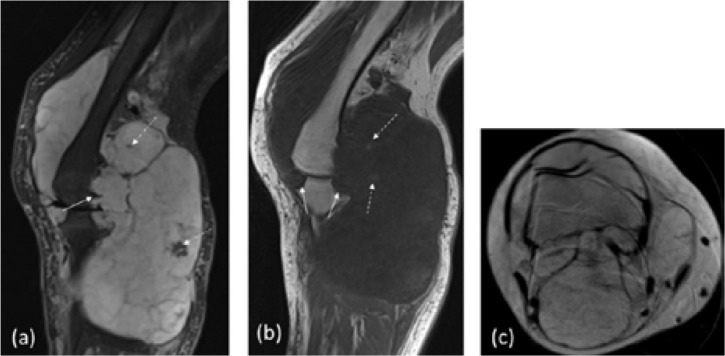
RIGHT knee MRI. Sagittal T2 fat sat (a), sagittal T1 WI (b), axial T2 WI (c). Significant distension of the knee joint capsule extending up into the suprapatellar region and down into the upper one-third of the leg. The material within the joint capsule shows internal signal intensity atypical for fluid, hyperintense on T2 and iso-to-hypointense on T1, with multiple internal heterogeneous septations. Multiple punctate low signal foci seen predominantly along the inferior aspect of the distended joint capsule with a faint T1 hyperintense component suggesting a haemorrhagic component [white dashed arrows in (a) and (b)]. Erosions along the anterior and posterior tibia and the posterior femoral condyle [white solid arrows in (a) and (b)].
